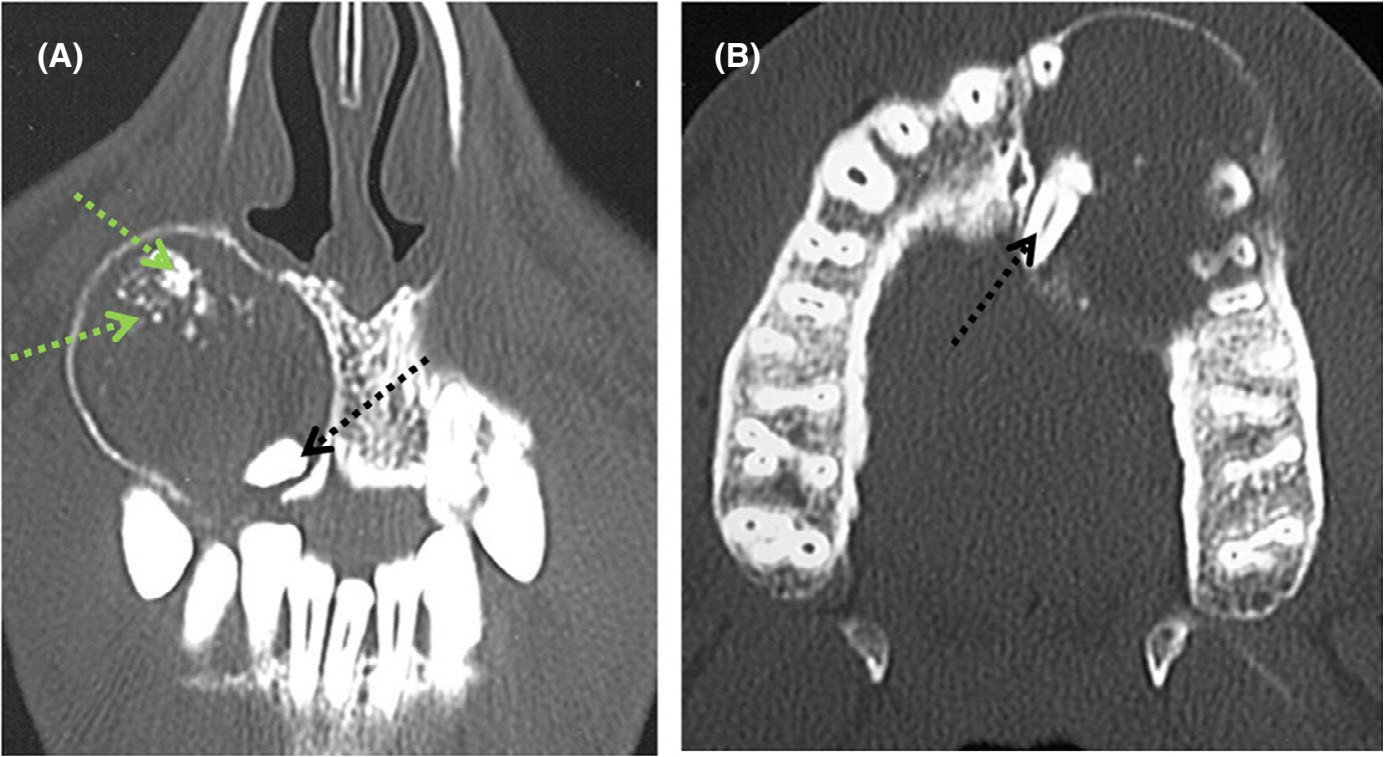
A, CT scan coronal slice in wider window revealing the presence of a mixed image pushing the external cortex, the bone palate, and the nasal fossae floor which are thinned, associated with the impacted 12 in full lesion. B, A CT scan axial slice showing a mixed image deforming the external and internal cortices, encompassing the apices of 11, 13, 14, and 15 with the presence of tooth 12 in full lesion
